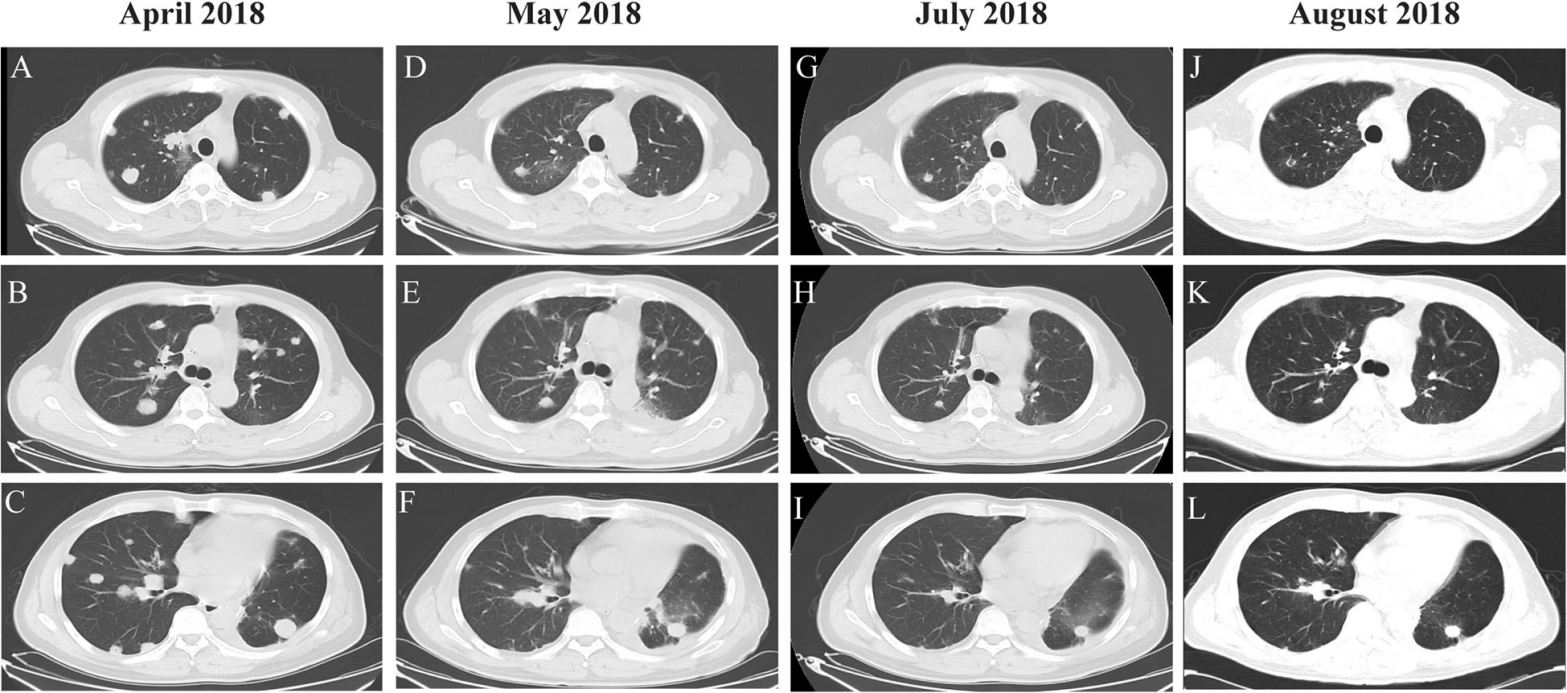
CT revealed a decreased size and number of lung nodules. Lung metastases in the entire lung were noted (a, b, c); CT scan after two cycles (d, e, f), four cycles (g, h, i) and six cycles (j, k, l) of pembrolizumab plus gemcitabine, the masses were markedly smaller and a marked response lasting for over 7 months
